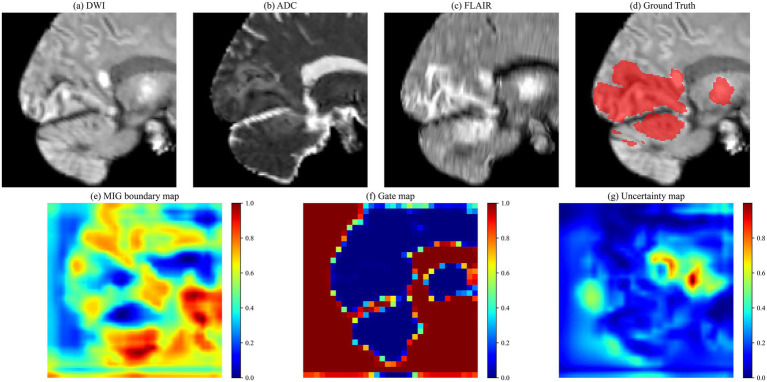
Multimodal images and intermediate response maps from the same case. (a) DWI, (b) ADC, (c) FLAIR, (d) ground-truth annotation, (e) MIG boundary response map, (f) deep gating map, and (g) uncertainty map. These panels provide a visual reference for the spatial relationship among lesion location, boundary response, gating intensity, and uncertainty.
